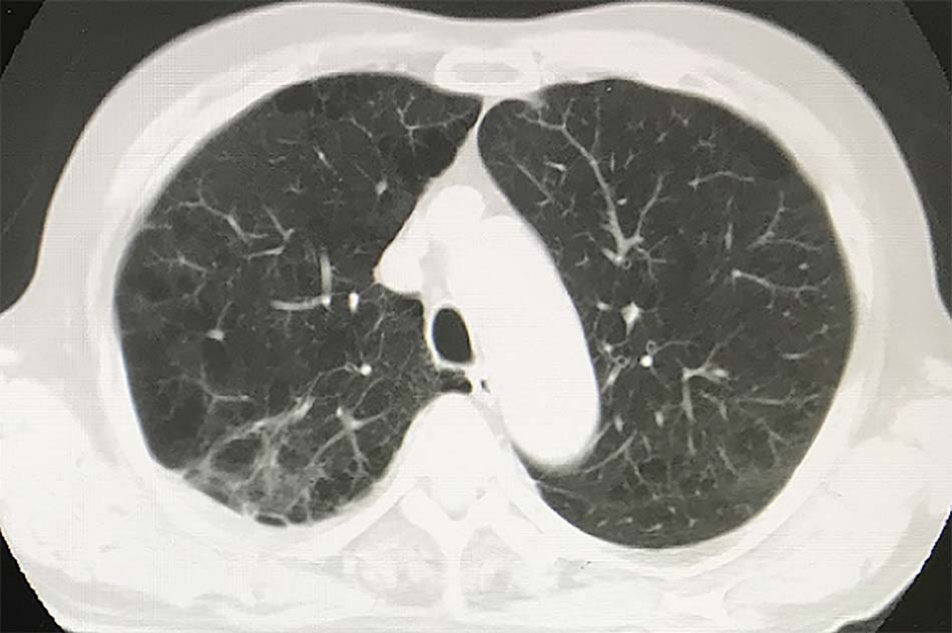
CT image of same patient as in Fig. 1 taken 27 months after treatment showed no evidence of disease.
